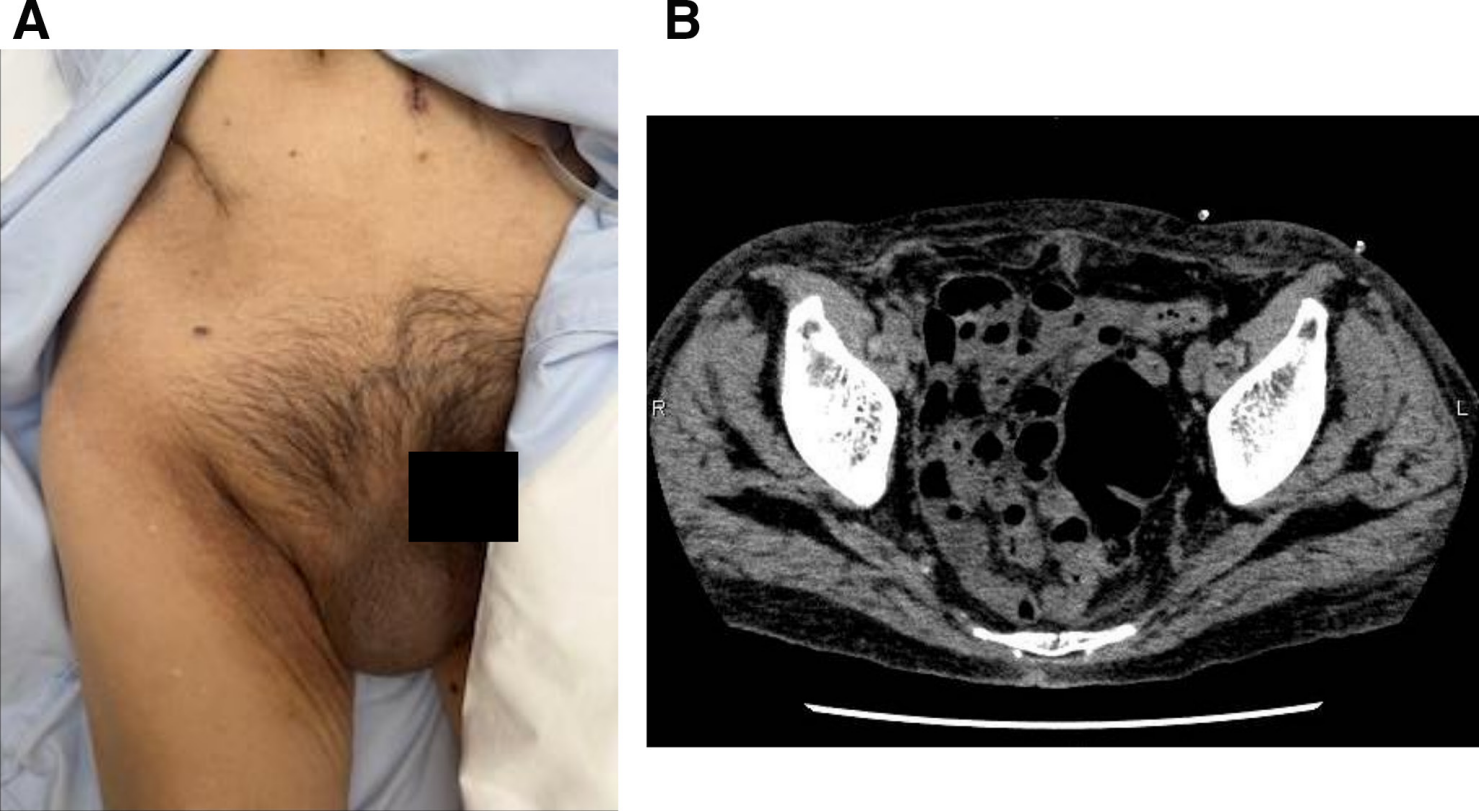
Postoperative findings at 6 months. (A) No inguinal swelling while standing. (B) No signs of recurrence on CT findings.
